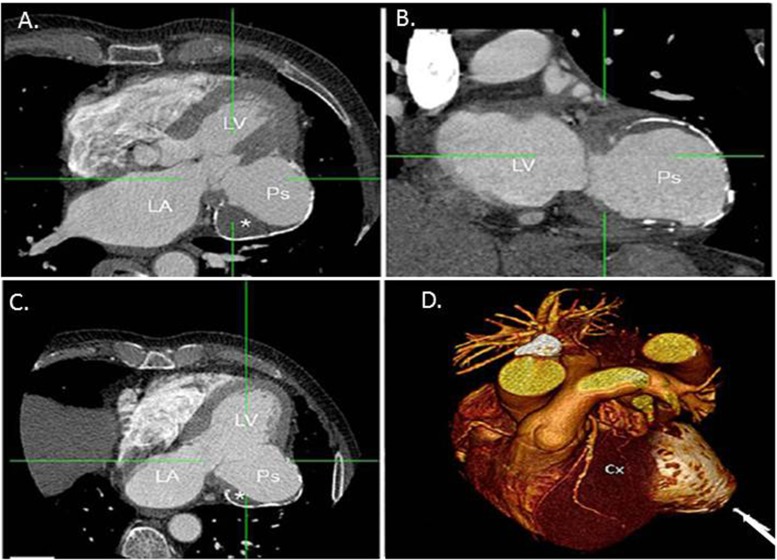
Left ventricular pseudoaneurysm. Contrast cardiac CT demonstrating a large pseudoaneurysm (Ps) of the left ventricular (LV) basal lateral wall as seen in oblique (a-c) and volume rendered (d) reformations. The patient had a history of a remote myocardial infarction that was complicated by cardiac tamponade due to left ventricular rupture. Note: thrombus (*) is visualized within the pseudoaneurysm and the rim of the pseudoaneurysm is calcified. LA, left atrium; Cx, circumflex coronary artery. Reproduced with permission from* [34]
